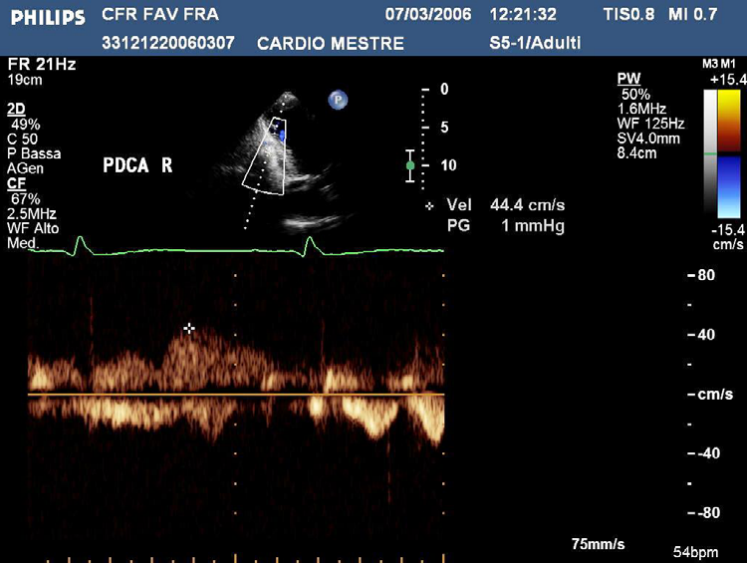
shows the Pulse Doppler envelope of the distal tract of left posterior coronary artery.
